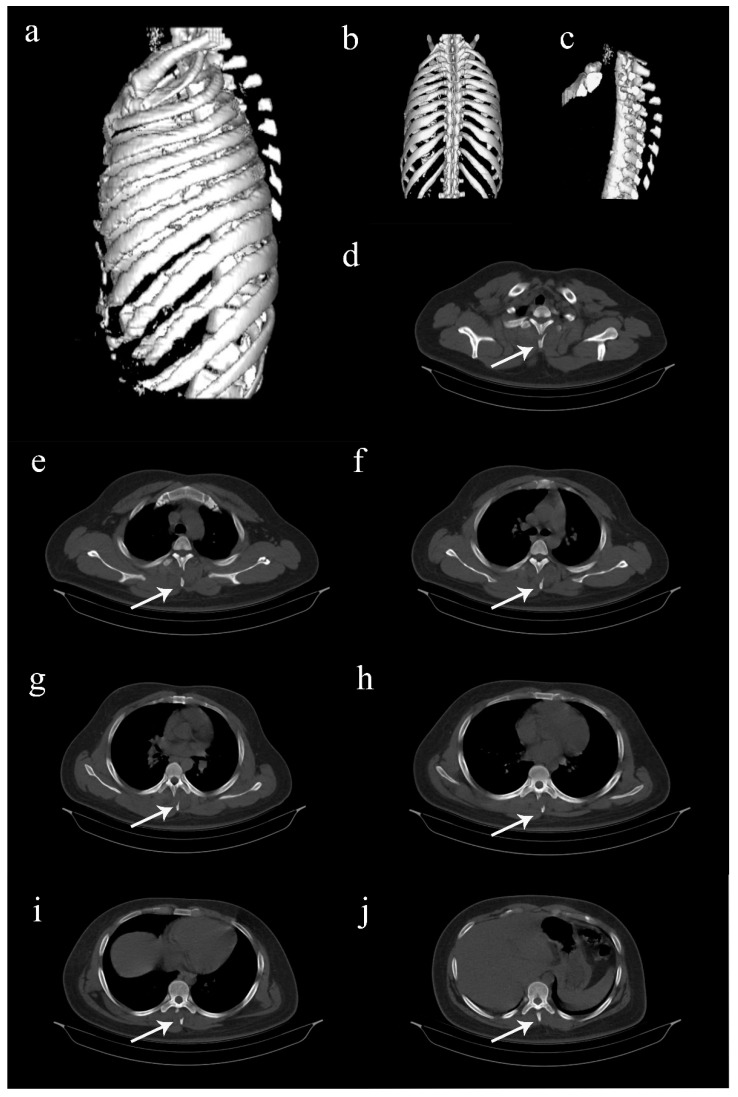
Computed tomography (CT) images of the thoracolumbar spine. (a–c) Three-dimentional reconstructed CT images showing multiple spinous process fractures extending from T2 to T8 levels, resembling clay shoveler’s fractures [1,2,3,4,5]. (d–j) Axial view CT images extending from T2 to T8 levels, showing the fractured spinous processes (arrows). Such involvement of multiple spinous processes in the thoracic spine is rare, and hence, sparsely reported [1,2,3]. Considering the stability of these fractures, it was decided to treat the patient conservatively. He was put on a thoracolumbar corset and was advised adequate bed rest for two weeks. Analgesics were provided, and self-care for the abrasions was taught. Protected weight bearing and mobilization exercises were started on the second week while on thoracolumbar corset support. The recovery was quick, and the patient was back to his full functional status by 6 weeks. Further follow-up was uneventful.
